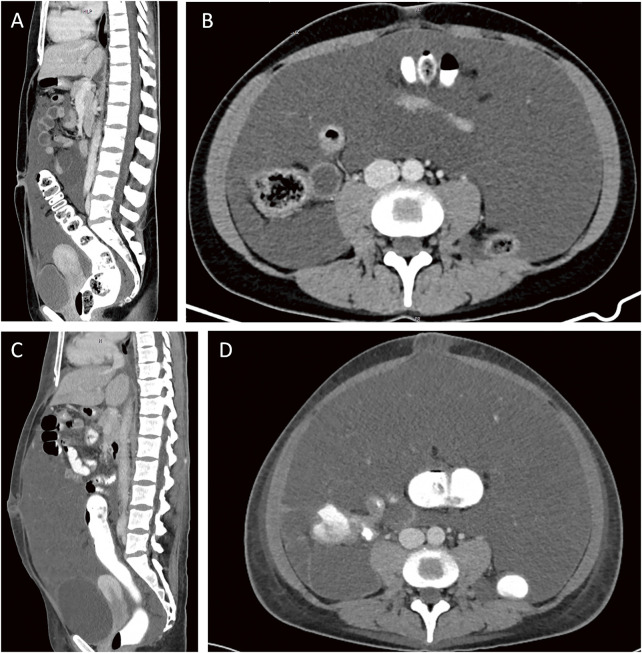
CT images 15 days (A, B) and 5 days (C, D) before surgery. CT, computed tomography
